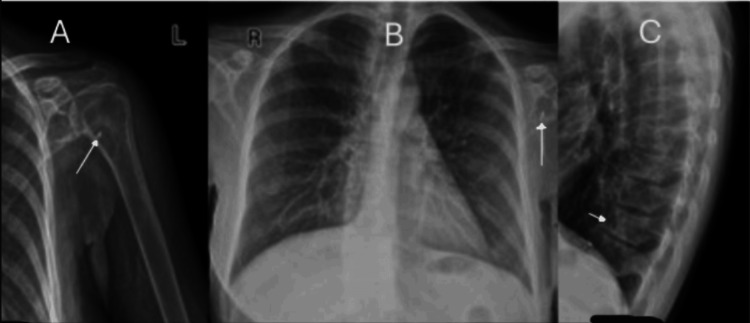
Skeletal survey radiographs showing sclerotic lesions (arrows) in the left humeral head (A), left scapula (B), and thoracic spine (T10-T12) and L1 vertebral body (C), consistent with metabolic bone disease in severe hyperparathyroidism.
